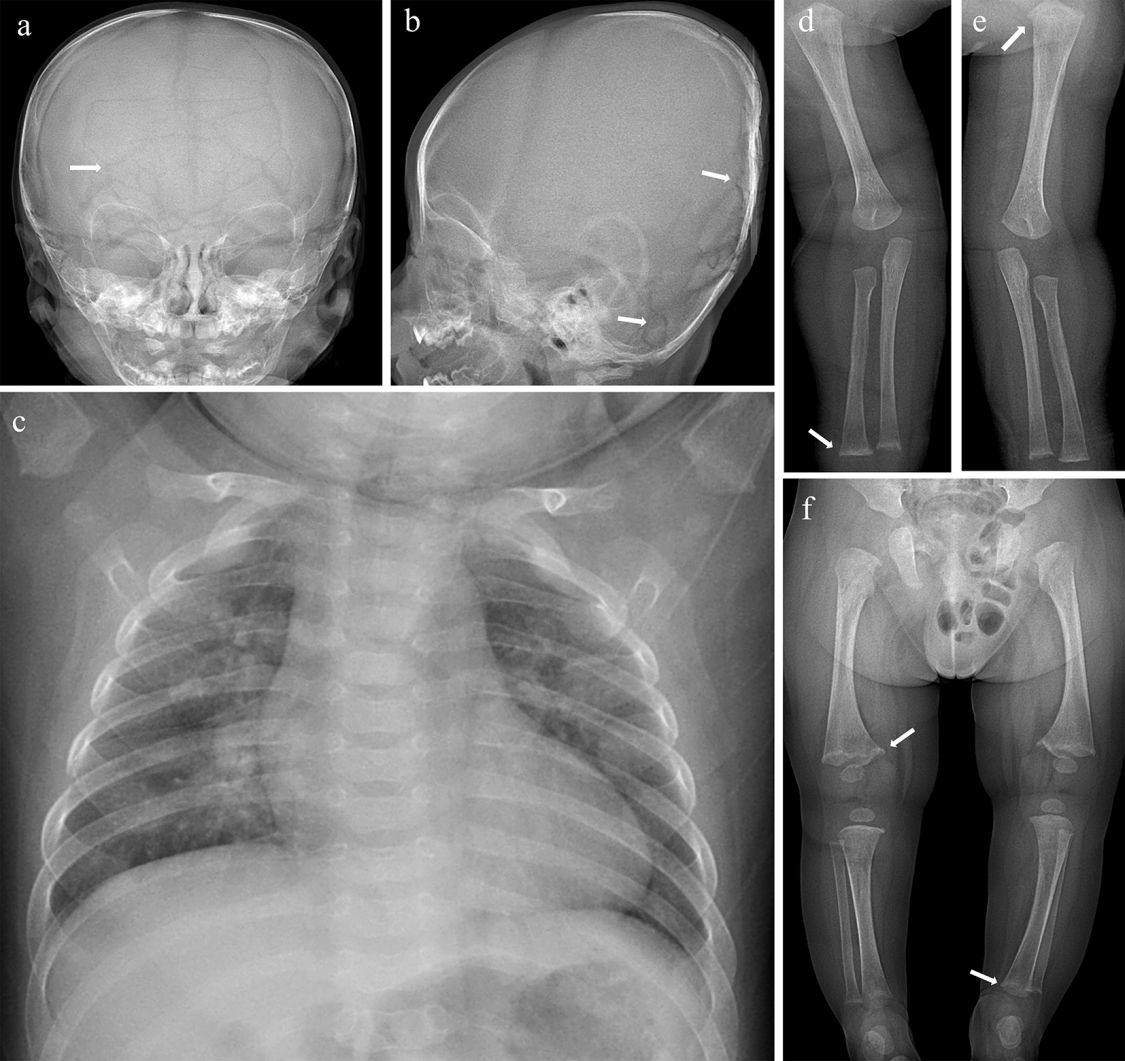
Plain radiograph images. (a-b) Anteroposterior and lateral skull radiograph shows numerous wormian bones along the sagittal suture, bilateral lambdoid sutures and mastoid fontanelle (arrows). (c) Anteroposterior chest radiograph shows flaring and expansion of anterior ends of bilateral ribs. (d-f) Enlargement and mineralization of metaphysis with lateral spurs involving right ulnar, left humerus, right femur and left tibia (arrows)
